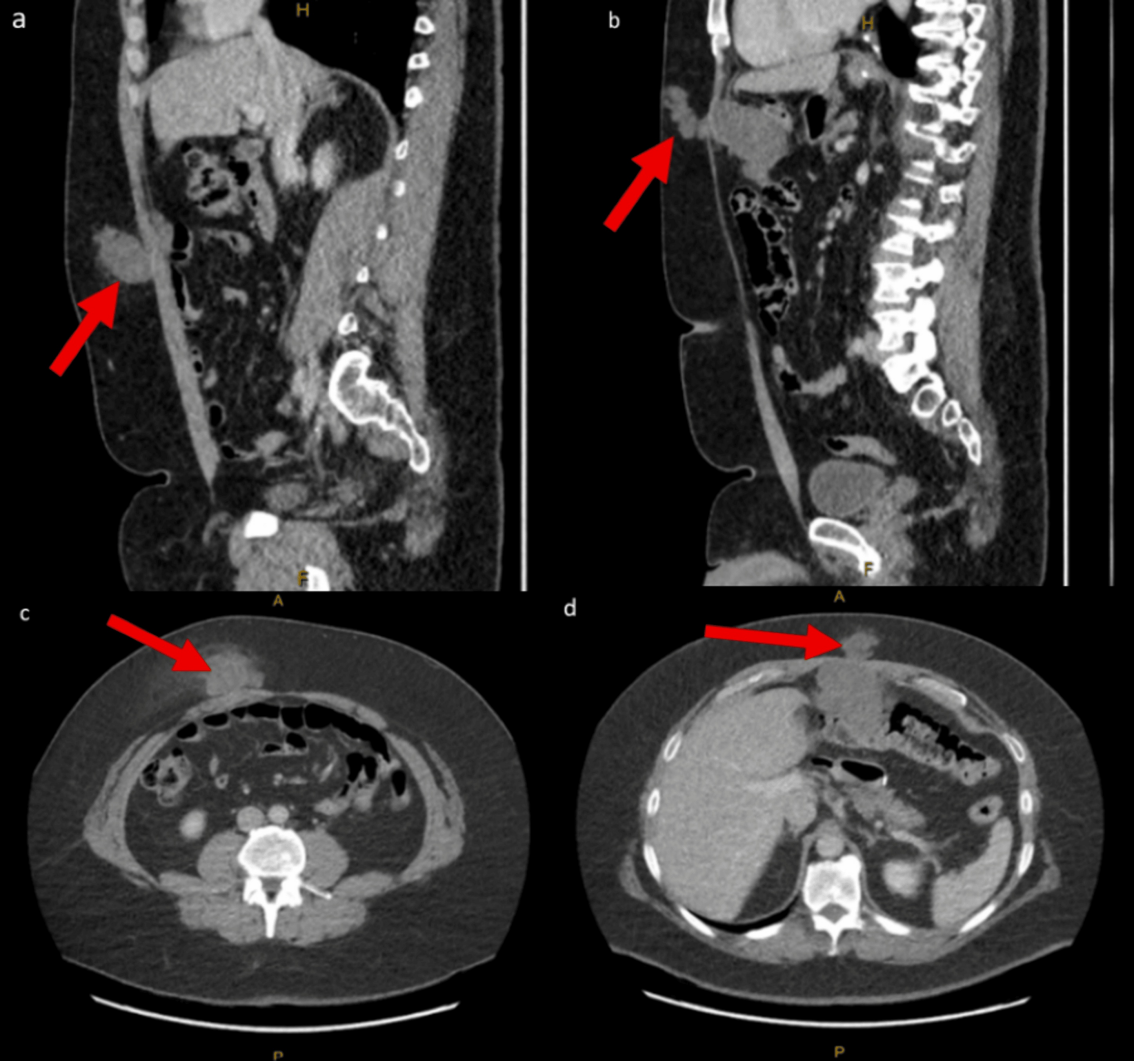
CT abdomen portal venous phase pre-operatively a: Sagittal slice with 2.99 mm thickness, W:370/L:40, demonstrating the inferior lesion (red arrow). b: Sagittal slice with 2.99 mm thickness, W:370/L:40, demonstrating the superior lesion (red arrow), which has an intraperitoneal extension. c: Axial slice with 5.0 mm thickness, W:475/L:35, demonstrating the inferior lesion (red arrow). d: Axial slice with 5.0 mm thickness, W:475/L:35, demonstrating the superior lesion which has intraperitoneal extension (red arrow).
